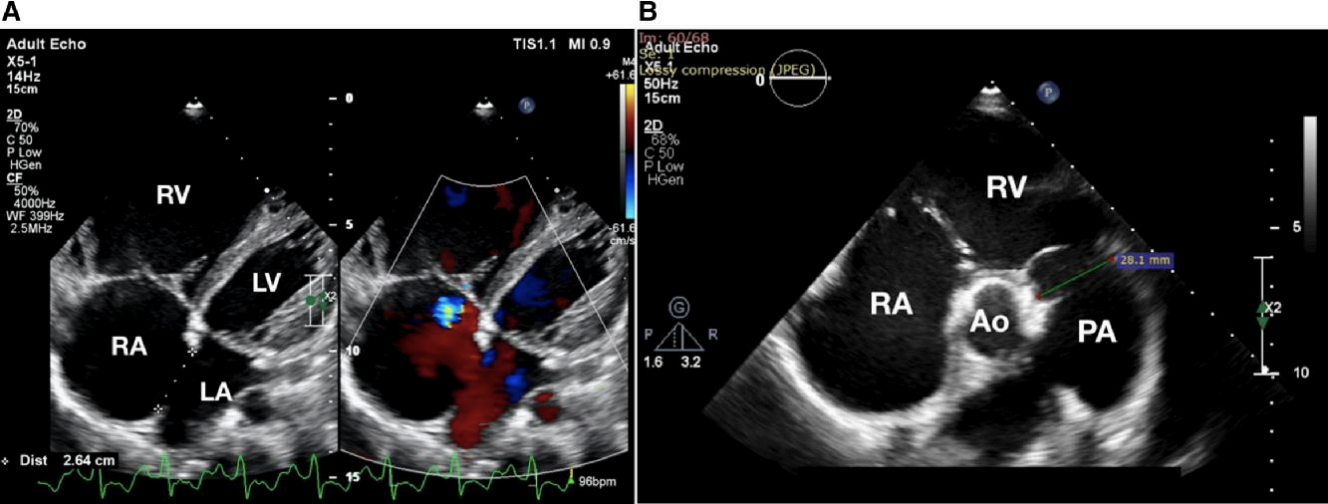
Echocardiography features in pregnant atrial septal defect patients. A transthoracic echocardiography showed a dilatation of the right atrium, right ventricle, main pulmonary artery (28.1 mm), and an oval-shaped 22 × 33 mm-sized secundum atrial septal defect, with an L-to-R shunt. (*A*) A four-chamber view. (*B*) A parasternal short-axis view. Ao, aorta; LA, left atrium; LV, left ventricle; RA, right atrium; RV, right ventricle.
